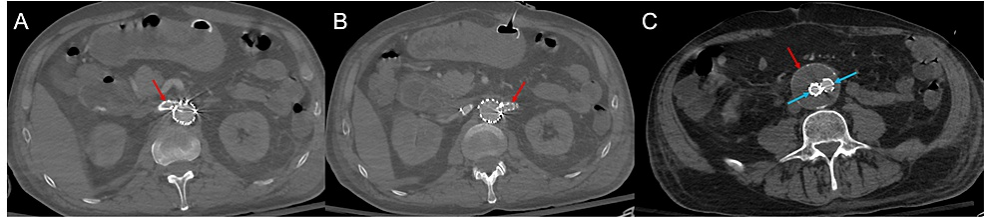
Surveillance computed tomography angiogram shows stent patency throughout various levels in the axial orientation. The right renal artery (A) and left renal artery (B) continue to demonstrate contrast patency (red arrows). A lower level in the axial orientation (C) shows the continued exclusion of the abdominal aortic aneurysm (red arrow) at the level of the common iliac arteries (blue arrows).
